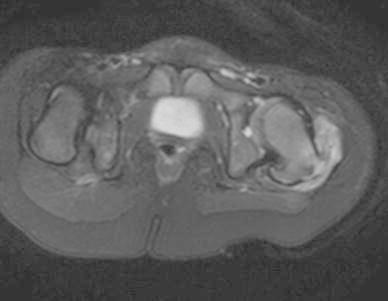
Sample good quality MRI image (T1 gadolinium). A chronic osteomyelitis of the left great trochanter was diagnosed
